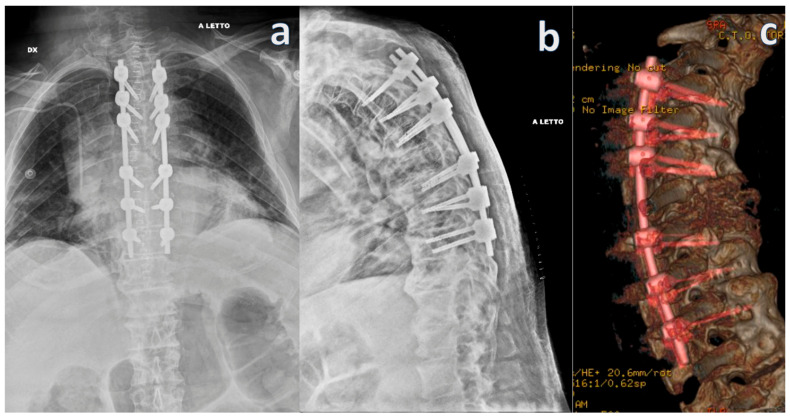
Surgical 3 + 3 stabilisation and decompression treatment in 70-year-old male patient affected by D7 fracture (AO type B) and spinal cord injury in ASD. (a) after surgery X-ray frontal view (b) after surgery X-ray lateral view (c) 3D CT scan.
